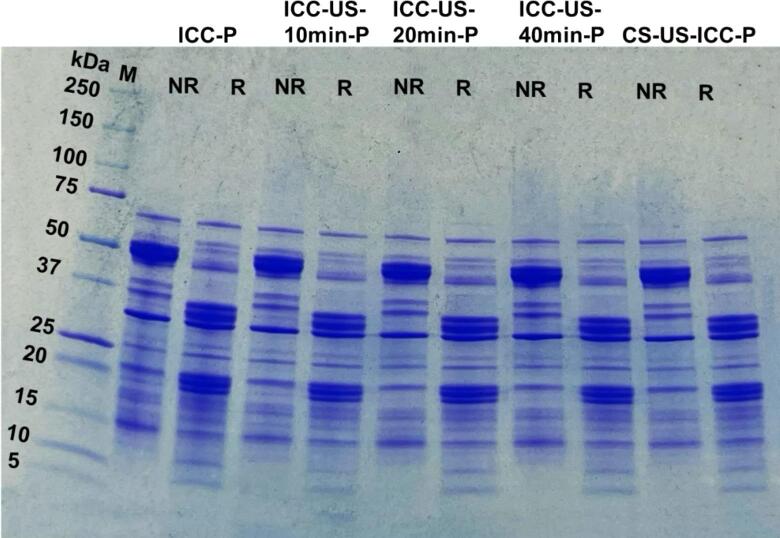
SDS-PAGE patterns of ultrasound treated chickpea cells protein isolates under non-reducing (NR) and reducing conditions (R) (ICC-P, protein isolated from intact chickpea cells; ICC-US-10 min-P, protein isolated from intact chickpea cells treated with 10 min ultrasound; ICC-US-20 min-P, protein isolated from intact chickpea cells treated with 20 min ultrasound; ICC-US-40 min-P, protein isolated from intact chickpea cells treated with 40 min ultrasound; CS-US-ICC-P, protein isolated from intact chickpea cells obtained from ultrasound treated chickpea seeds.).
